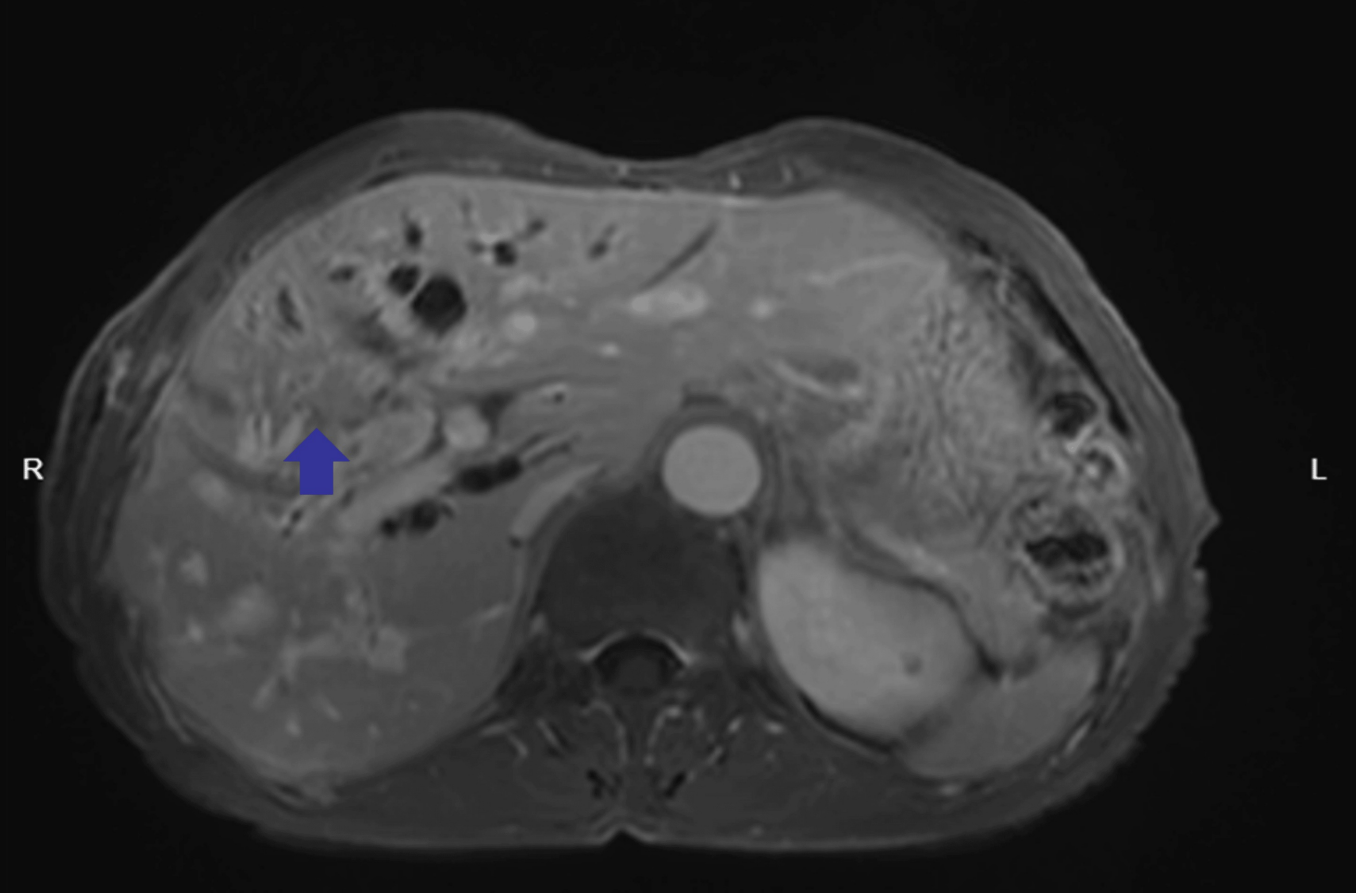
Contrast-enhanced MRI Contrast-enhanced MRI demonstrates a nodular lesion in the hepatic hilar region, showing slightly long T1 and T2 signals, slightly hyperintense signal on fat-suppressed imaging, measuring approximately 26 mm × 23 mm, with ill-defined borders (blue arrow).
